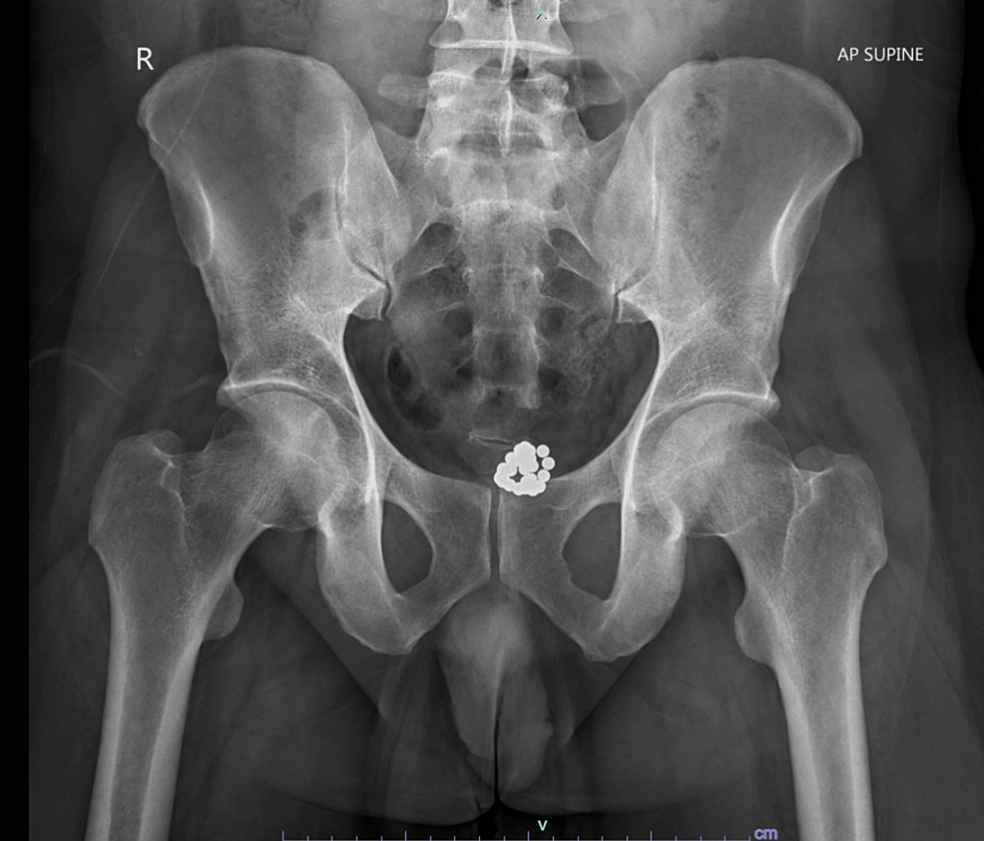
Pelvic X-ray (anteroposterior view): Presence of magnetic balls in the bladder.
